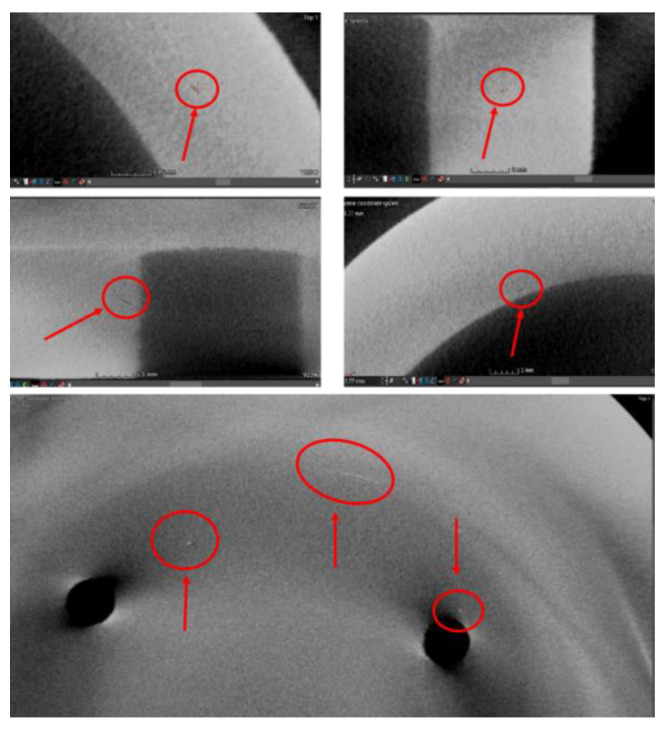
Representative X-ray CT scans for one closed impeller built at B + 60° orientation.
